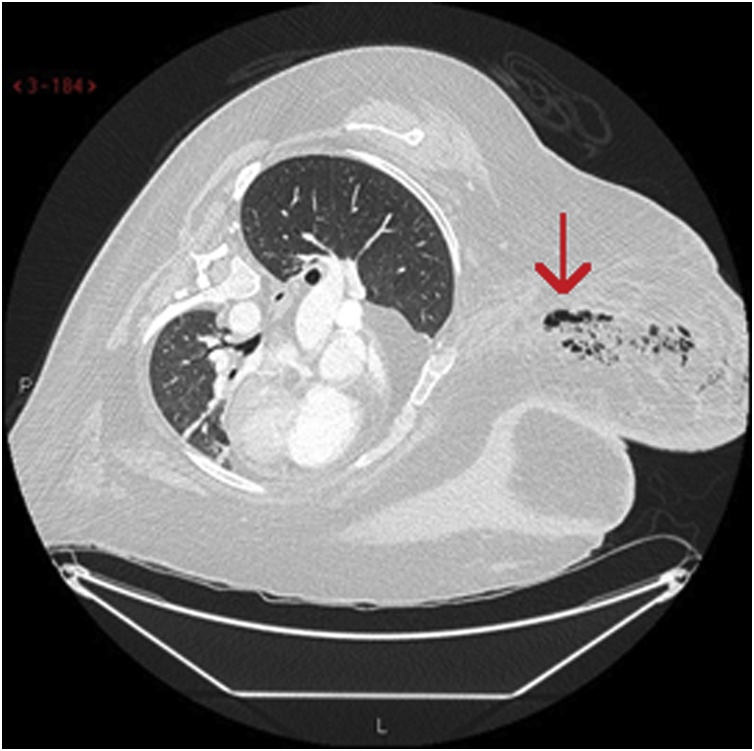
CT imaging of right breast demonstrating extensive soft tissue gas.
